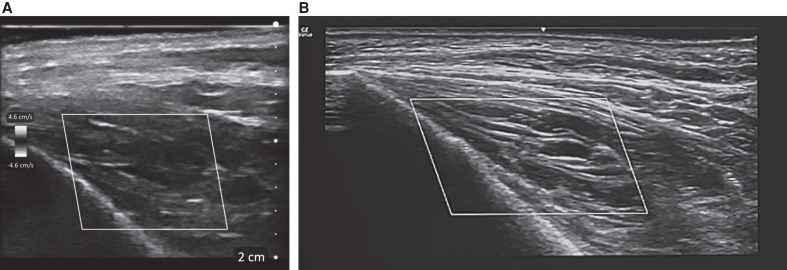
Comparison of a handheld ultrasound device. (A) Clarius L20 (Clarius Mobile Health, Vancouver, BC, Canada) and a high-resolution medical ultrasound system. (B) GE Venue Go, L4-20 probe (GE Healthcare, Chicago, IL), demonstrating differences in axial and lateral resolution.
